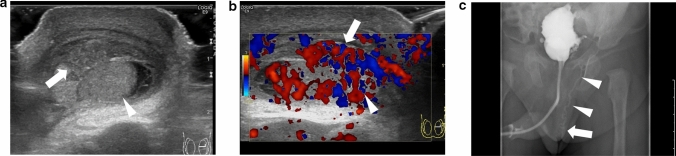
Ultrasonographic images of a 2-month-old baby with acute epididymitis with orchitis. a Swelling of the epididymis and edematous changes in the scrotal dermis are evident. b Hypervascularity of the epididymis and testes can be visualized. Epididymitis with orchitis was diagnosed based on these findings. c Voiding CUG shows reflux into the spermatic cord and epididymis
